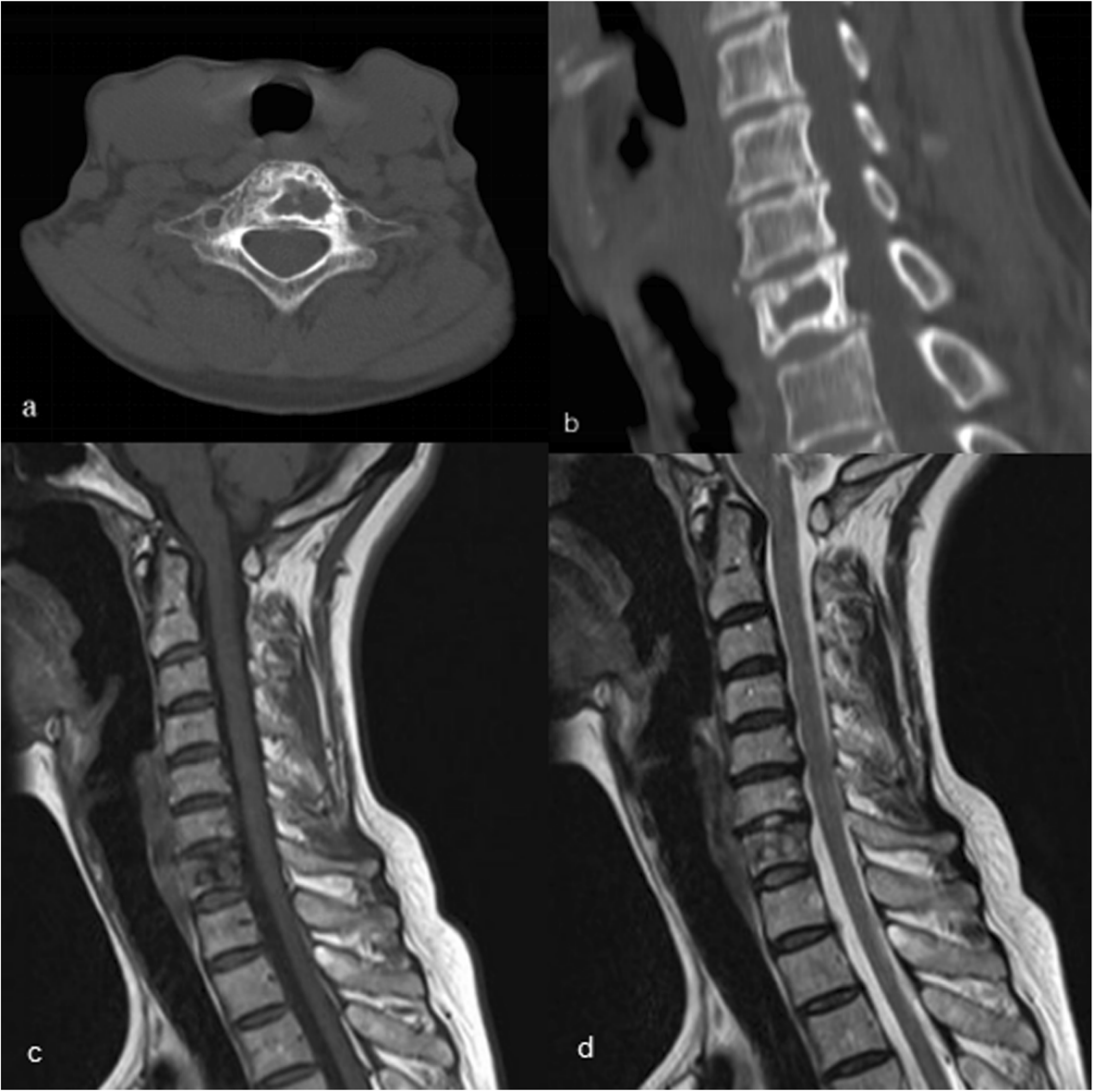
Preoperative imaging of the patient with cervical fibrous dysplasia. a, b CT images demonstrated low density of the seventh cervical vertebra, with high-density hardening visible around the edges. c, d MRI scans of the cervical spine indicated an expansile lytic lesion with isointensity on T1-weighted imaging and hyperintensity on T2-weighted imaging
